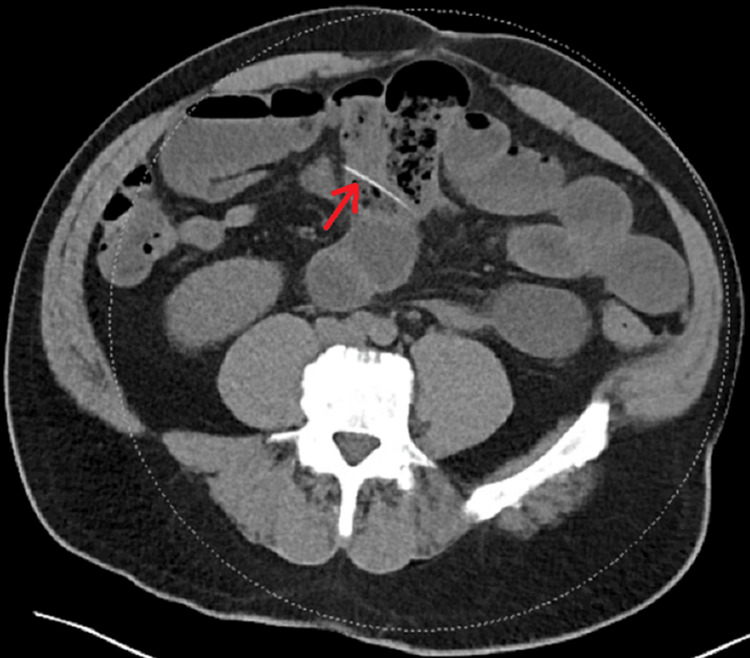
Axial non-contrast CT imaging of the abdomen and pelvis demonstrating the linear hyperlucent object appearing to lie within a loop of the small bowel.
